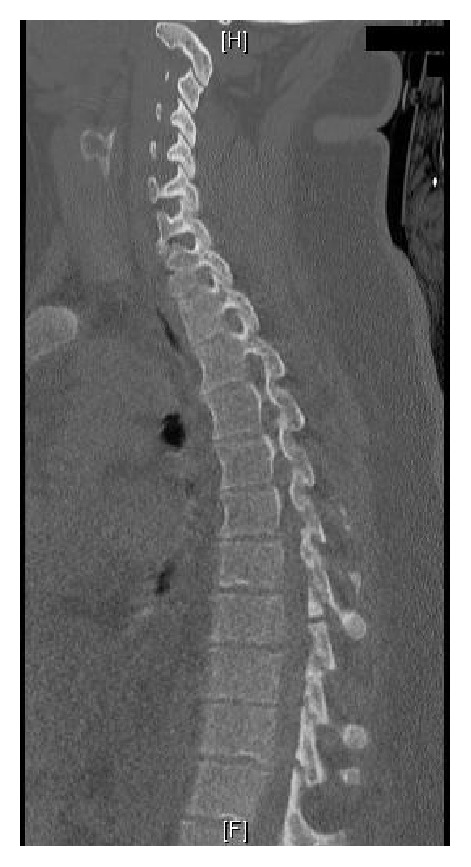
CT of the thoracic spine on presentation to the ED, showing a fracture through the lamina of T8.
